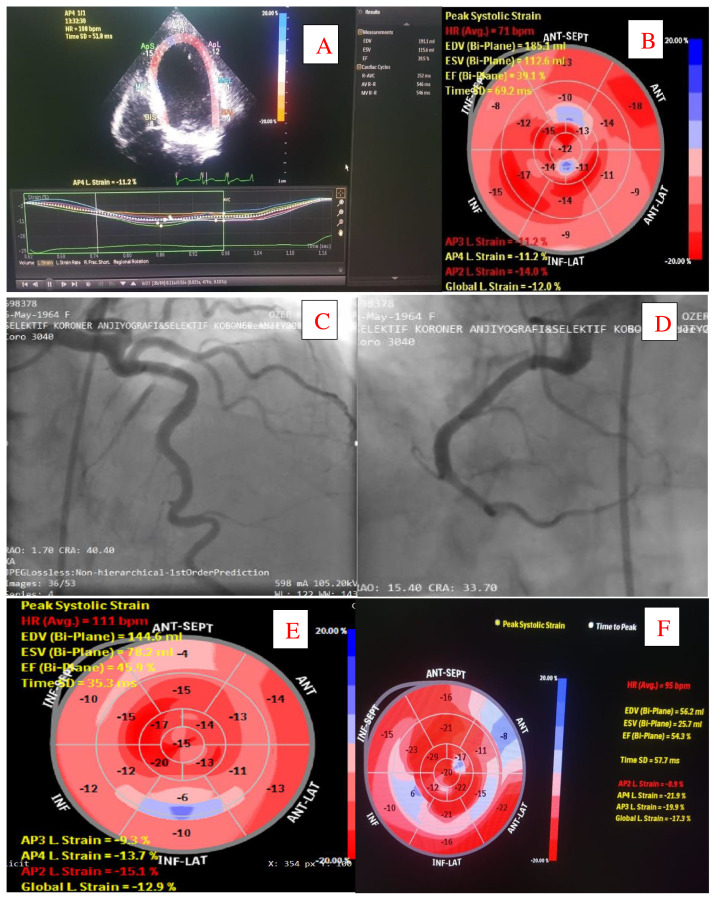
A patient with a transient decrease in systolic function. Echocardiographic imaging showed diffuse left ventricular hypokinesis and left ventricular dysfunction (LVEF= 39.1%) (A and B). She was treated with antiviral drugs (favipiravir), chloroquine, medical treatment for heart failure, and high flow oxygen therapy with progressive clinical stabilization. Normal coronary arteries were detected in coronary angiography (C and D). High-sensitivity troponin T levels were within normal limits in follow-up. Echocardiography follow-up revealed that LVEF was 45.9% on the eighteenth day ( E ) and 54.3% on the thirtieth day (F) after clinical improvement begins.
